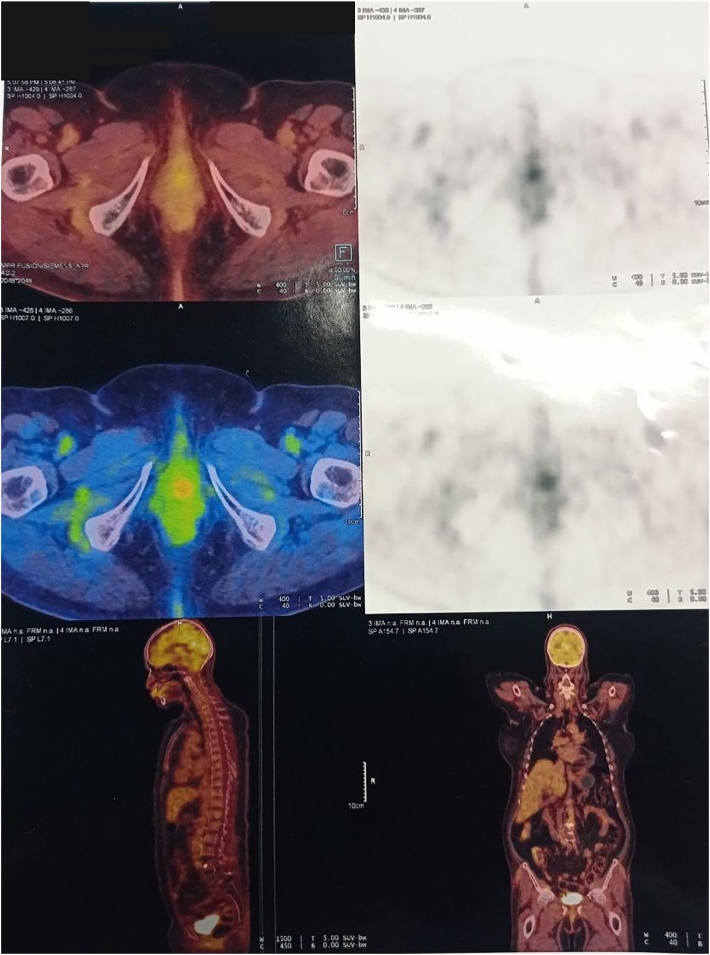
FDG PET-CT: FDG uptake in the distal part of the vagina.
